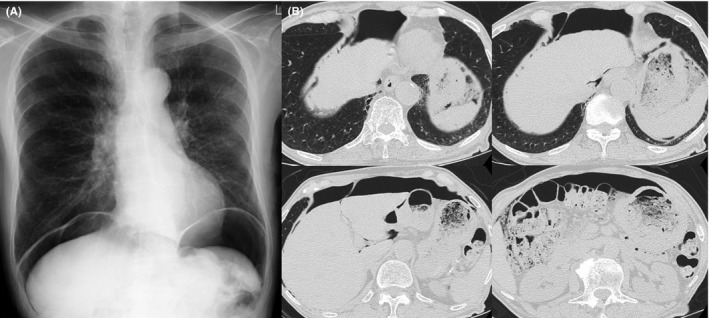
Radiological examination of Case 2. A, Chest X‐ray showed free air beneath both of the diaphragms. B, Computed tomography showed remarkable pulmonary emphysema and a large amount of intra‐abdominal air
